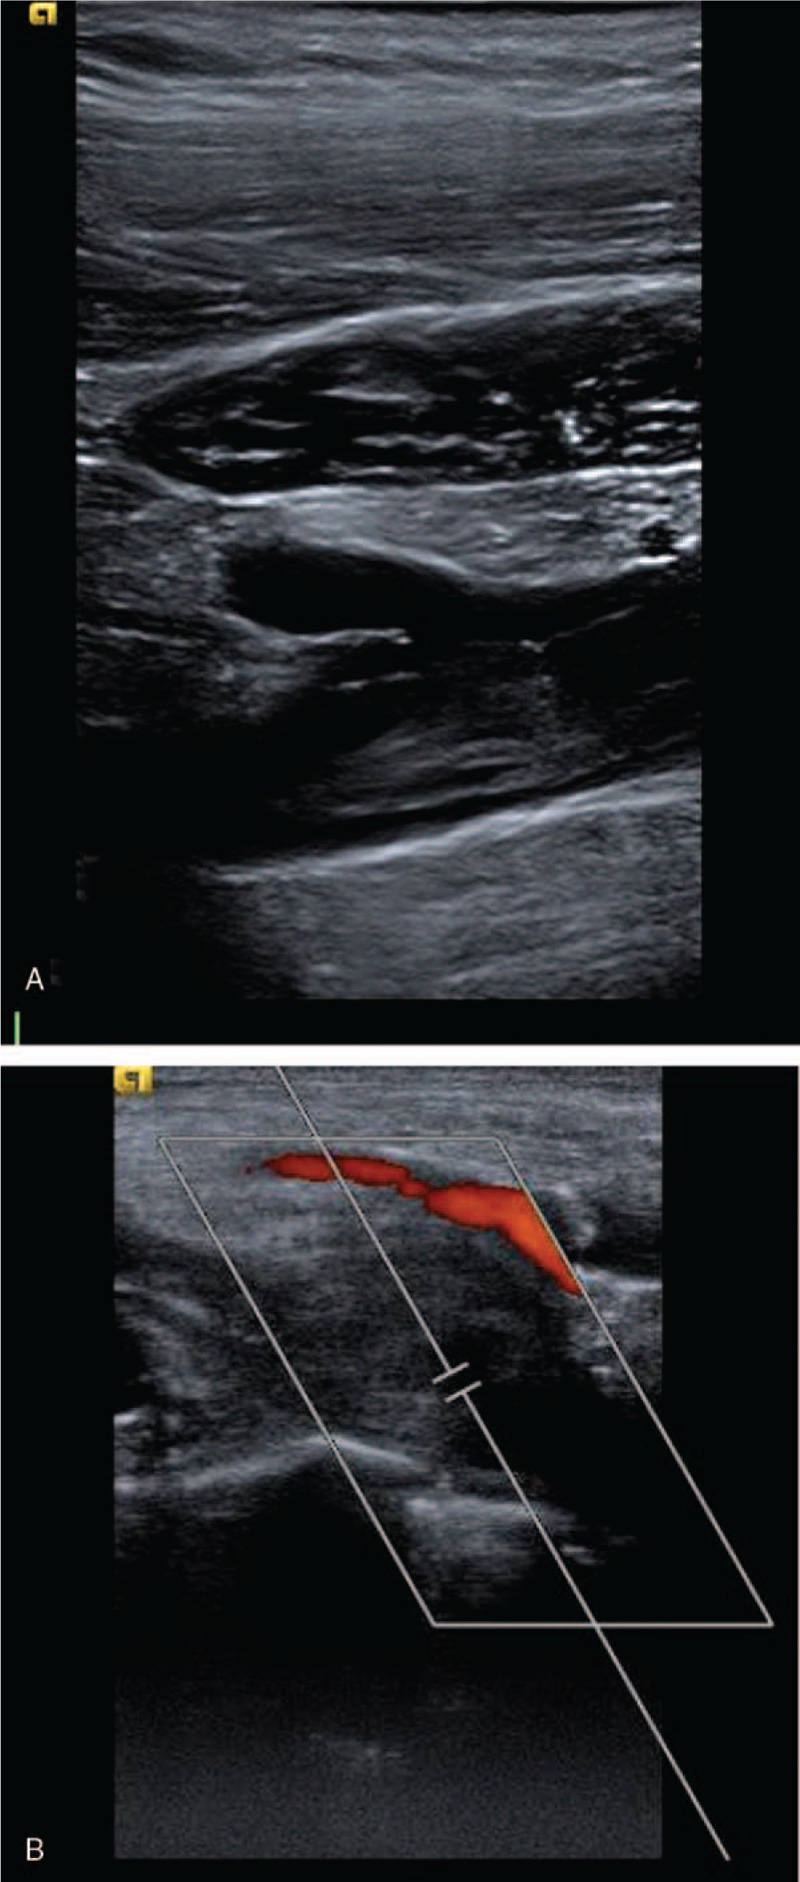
(A, B) Ultrasound duplex demonstrating occlusive right axillary and subclavian venous thrombosis for case 1.
